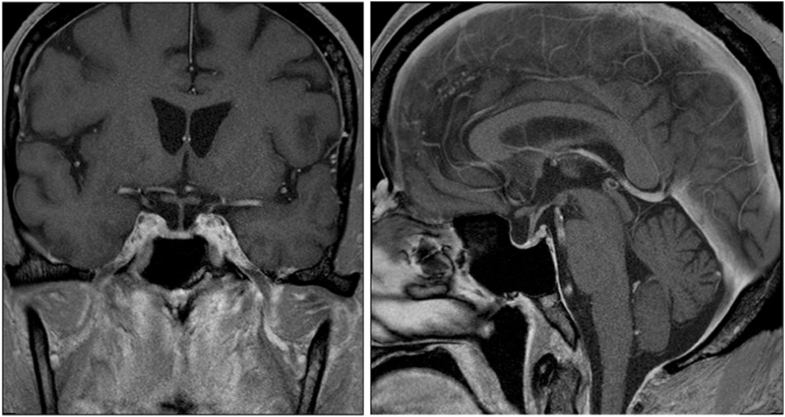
Magnetic resonance imaging (MRI) of the pituitary without/with contrast at the time of the third cyclical episode of Cushing disease. The MRI showed a partially empty sella with no evidence of a pituitary mass. Left, coronal view. Right, sagittal view.
